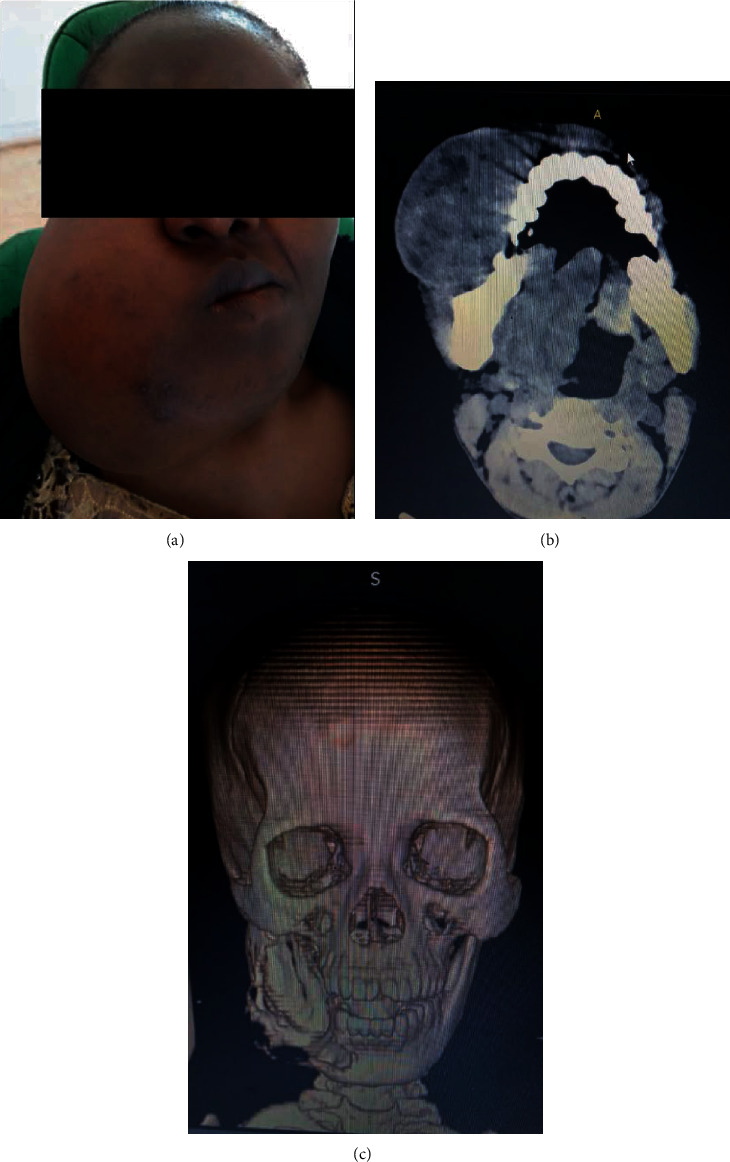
Clinical image of a 43-year-old female patient diagnosed with a *EWSR1*-rearranged clear cell odontogenic carcinoma of the mandible (a), CT scan axial view (b), and 3D-refomatted CT scan showing an osteolytic destructive mass in the mandible with thinning and perforation of the cortical border of the mandible (c).
